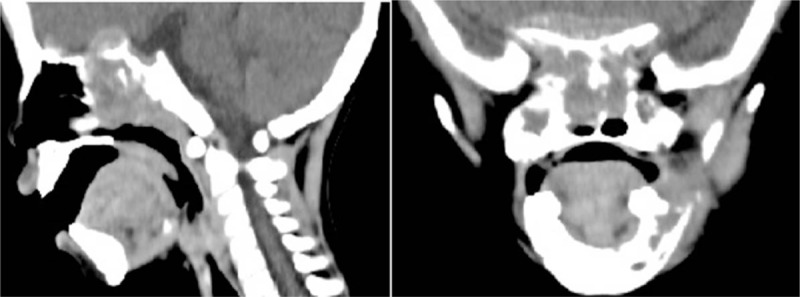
HRCT image showing skull base neoplasms at the nasal cavity and nasopharynx with bone metastasis.
